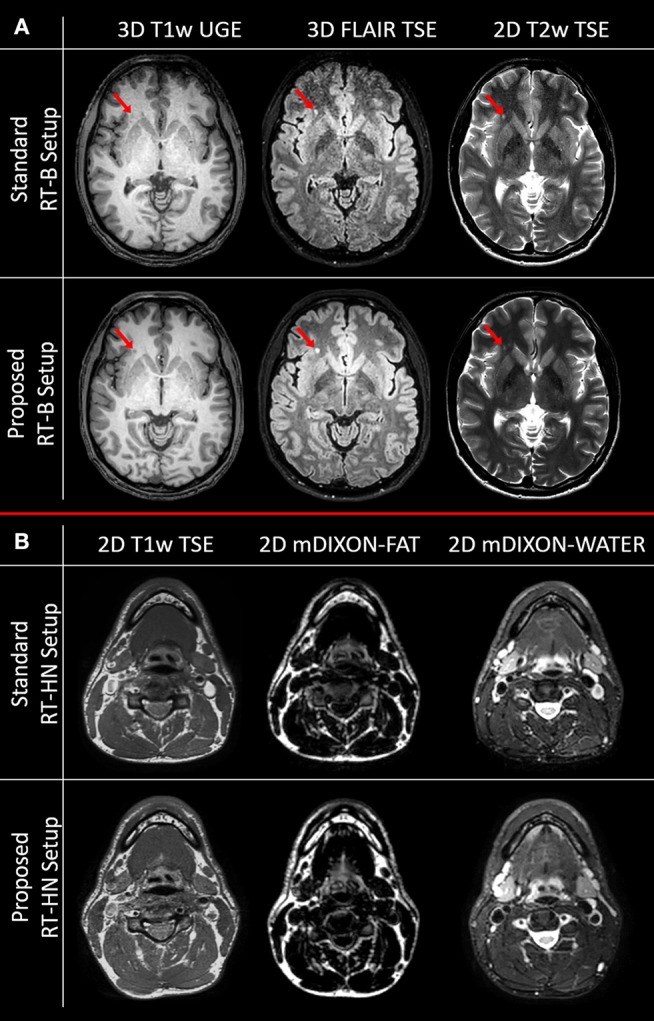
Image quality comparison for clinically acquired brain (A) and head-and-neck (B) MRI sequences for the standard and the proposed setups. The red arrows indicate a white matter lesion.
